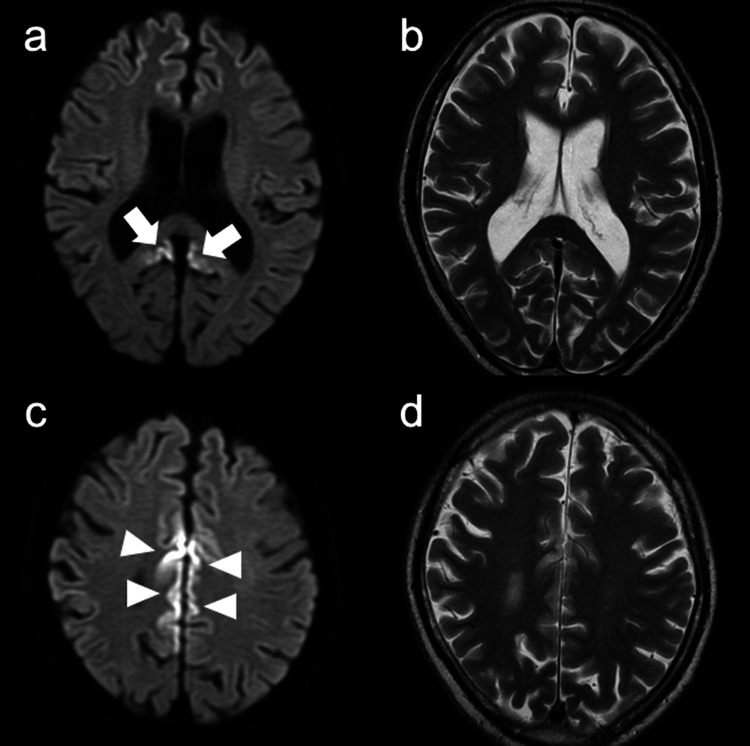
Brain magnetic resonance imaging (MRI) findings at two months before referral. Brain MRI reveals increased signal intensity in the cerebral cortices with swelling on diffusion-weighted images (A, C) and T2-weighted images (B, D) (arrows and arrowheads).
